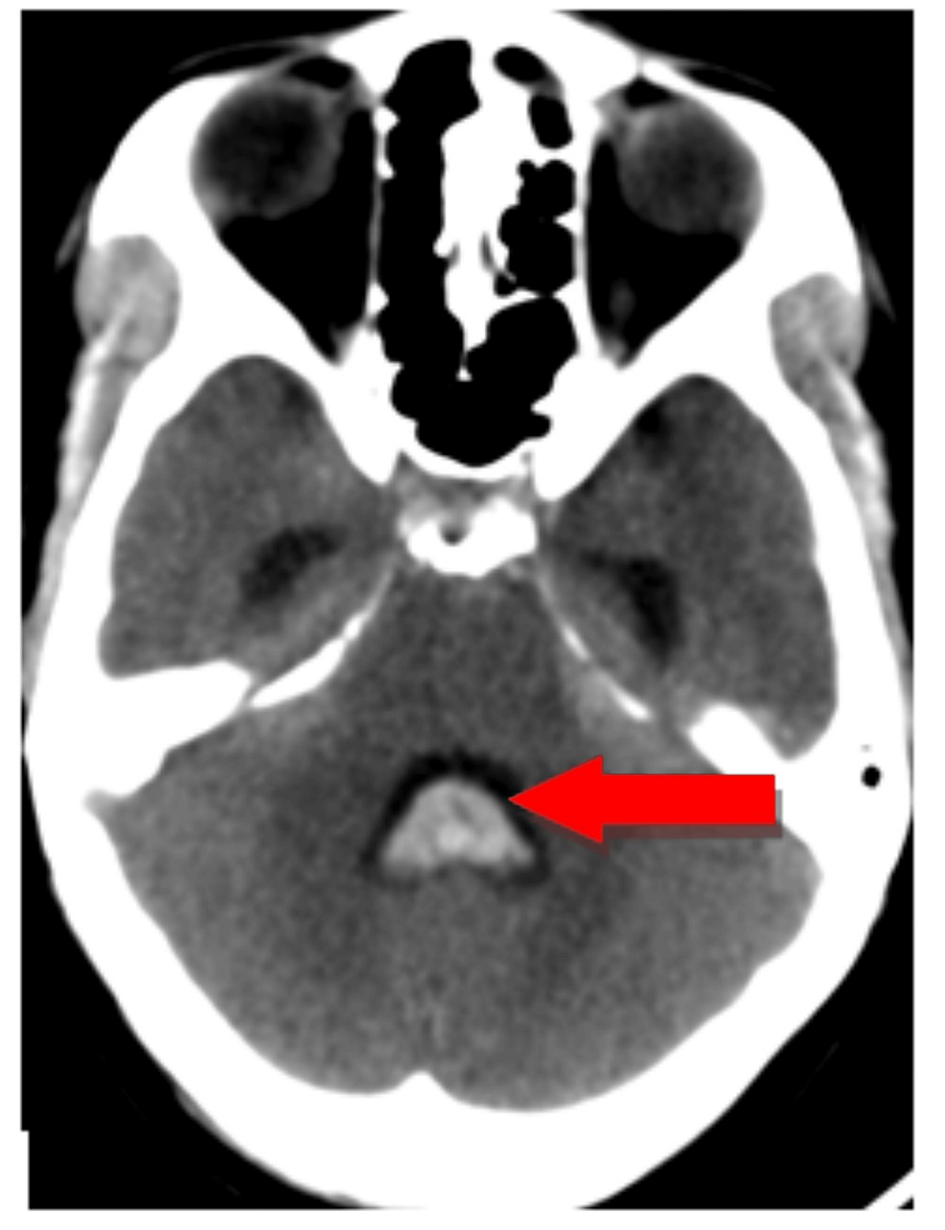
Axial non-contrast CT brain showing haemorrhage in fourth ventricle (red arrow) with extension into posterior subarachnoid space causing obstructive hydrocephalus.
